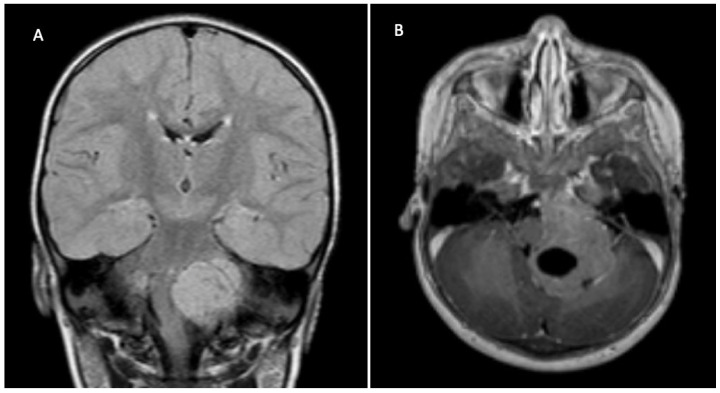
11 months old girl with swallowing difficulties and facial palsy. FLAIR MRI (A) and contrasted T1 MRI (B) shows enhancing tumor invading the cerebellum from the left cerebellopontine angle. Diagnosis: anaplastic ependymoma, PFA-type. Therapy: after NTR chemotherapy (HIT-MED Therapy Guidance modified SKK) and proton-beam irradiation, early local recurrence during adjuvant treatment and re-resection, 3rd look surgery for tumor residual after radiation therapy termination.
